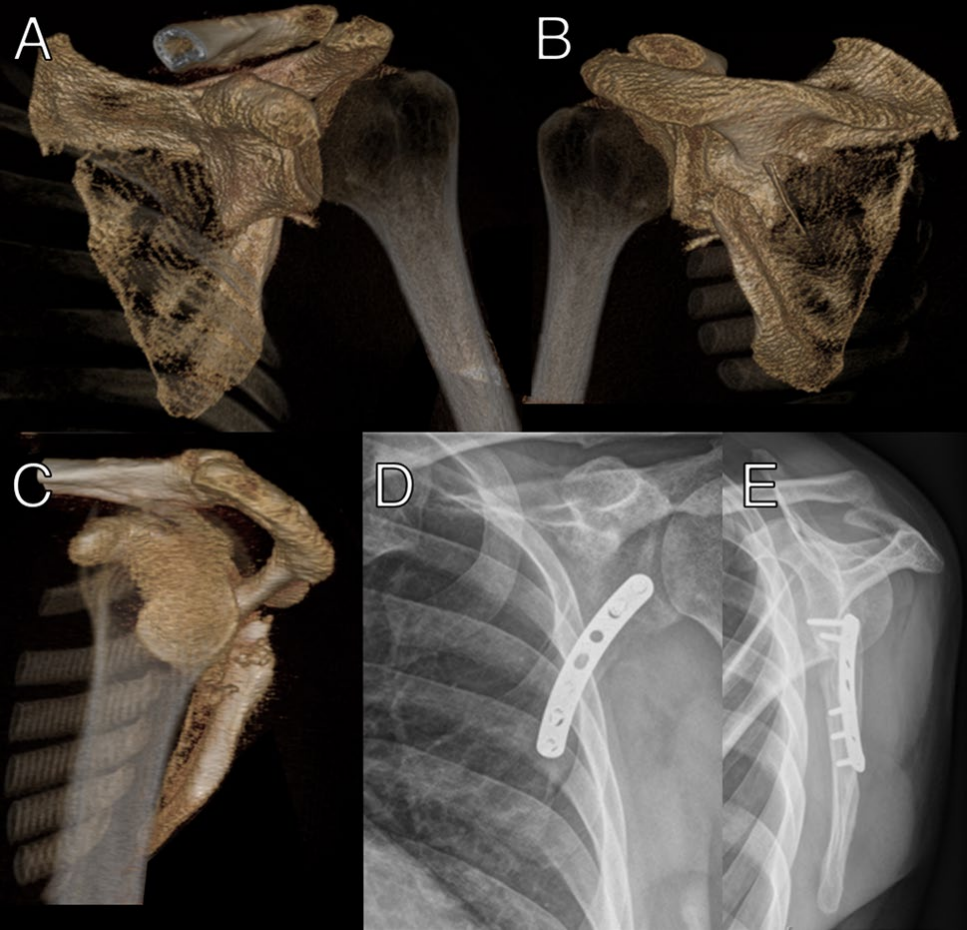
A–E: Preoperative 3D reconstruction showing a scapular body fracture with coracoglenoid block formation (A–C). Postoperative anteroposterior (D) and Y-view (E) radiographs following ORIF
